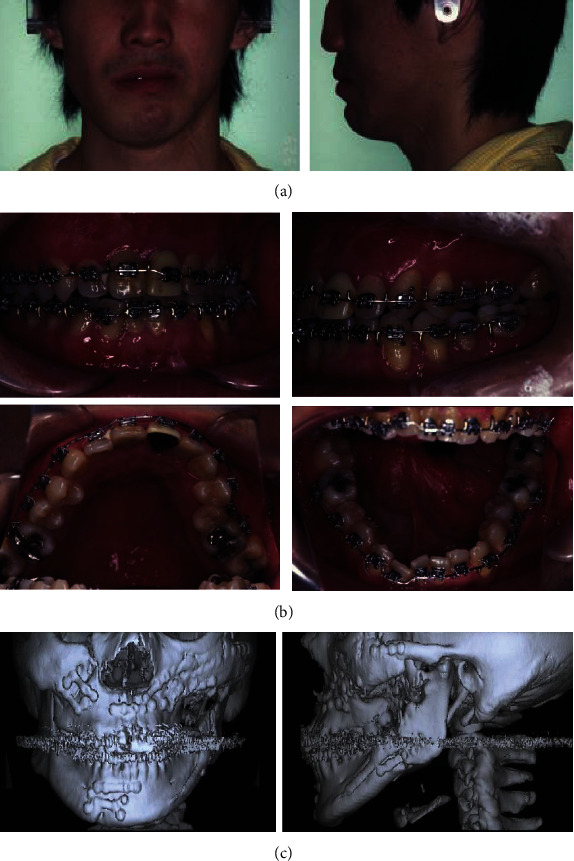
Facial (a) and intraoral photographs after 9 months (b) and cephalic CT images after open reduction (c).
